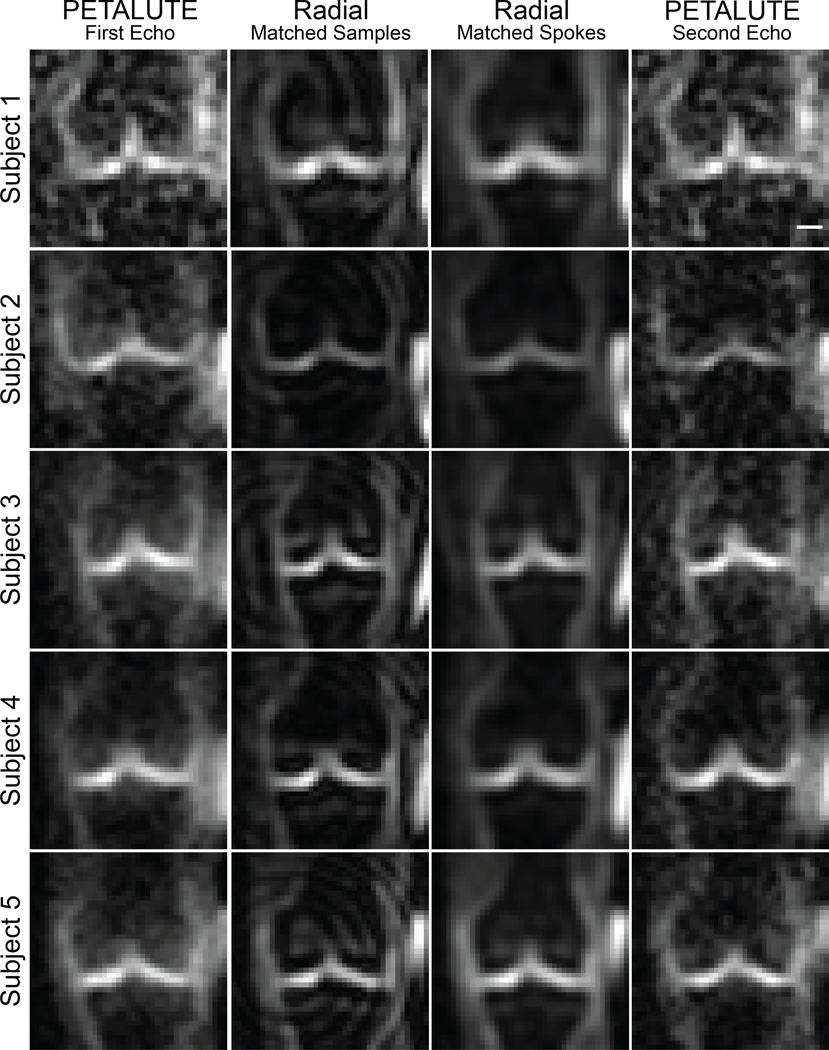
^23^Na MRI of the knee was implemented using PETALUTE and DA-Radial UTE. Cuboid cropped volumes were centered on the joint midline and include the distal femur and proximal tibia. Coronal slices centered on the joint space are shown for each subject and UTE acquisition. Scale bar = 1.5 cm
